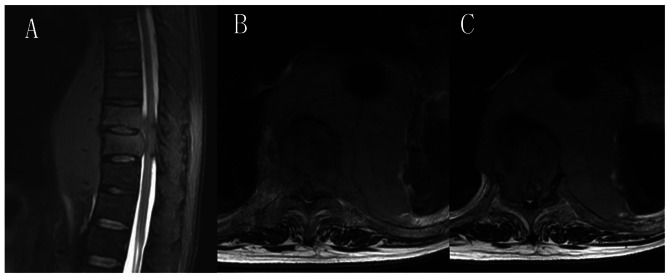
(A) The sagittal T2-weighted magnetic resonance imaging (MRI) showed a large lytic mass of the T7-T12 region with extraosseous extension. (B-C) MRI showed extradural spinal compression by a tumor mass (T9 and T10) with tumoral involvement of the entire bone marrow in T9 and T10, respectively.
